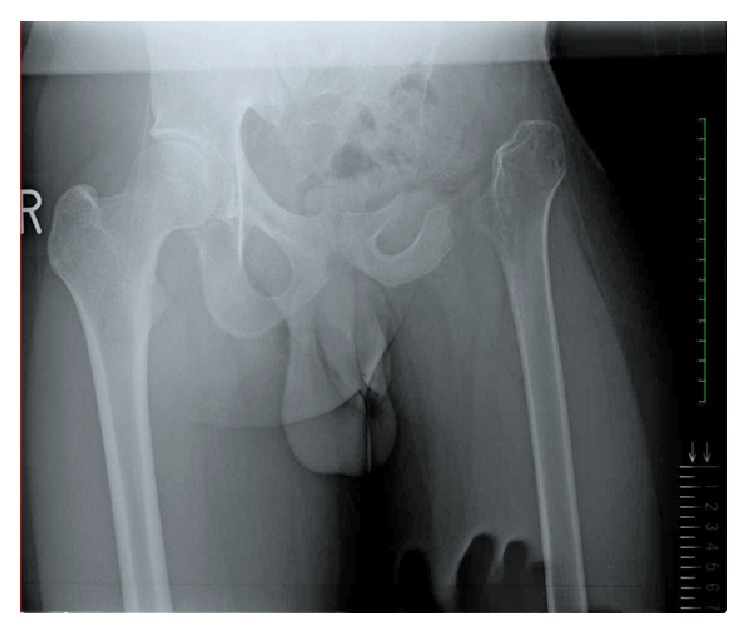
Radiograph of the hip joints at first visit.
